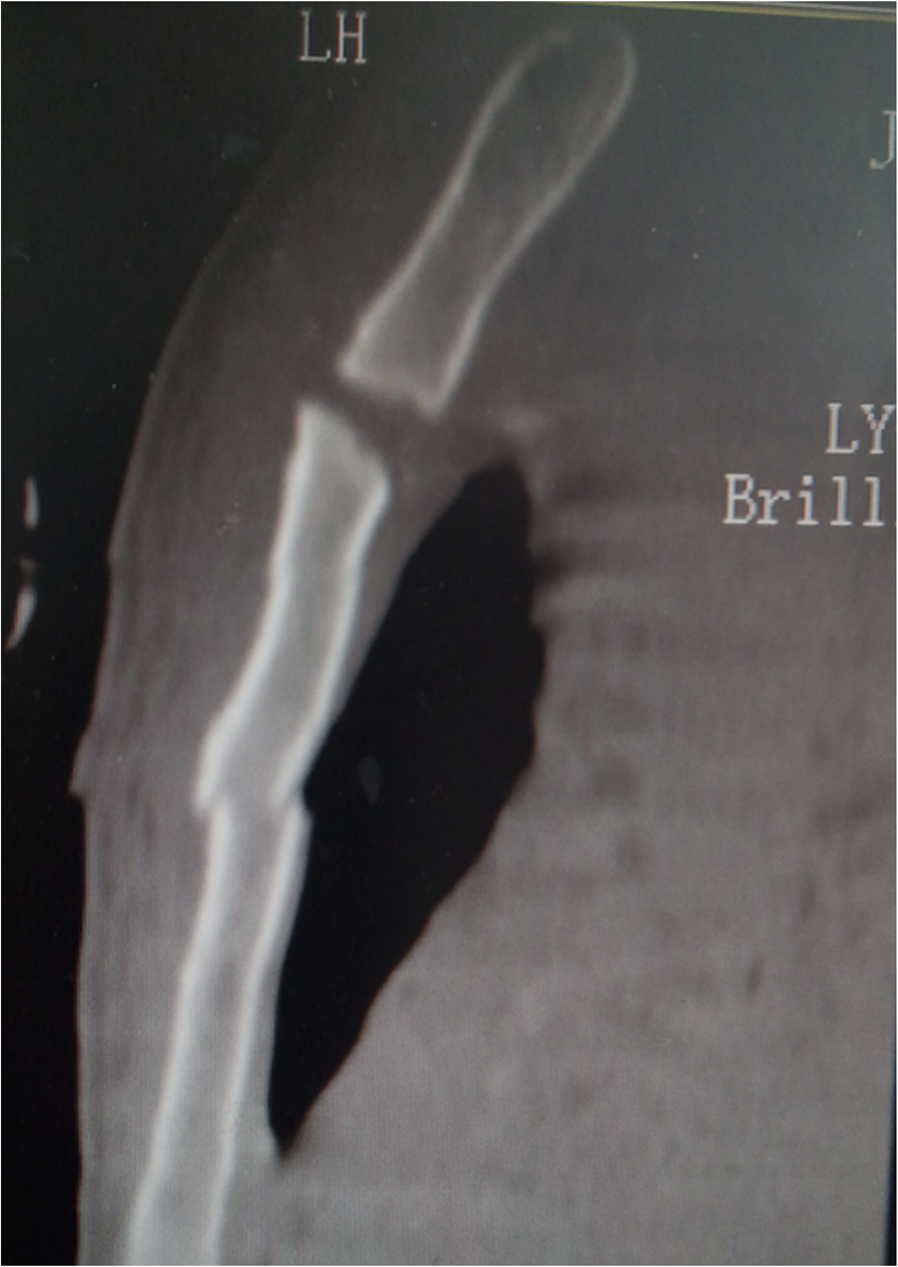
Sagittal CT scan of the sternum showing the sternal fracture without dislocation
